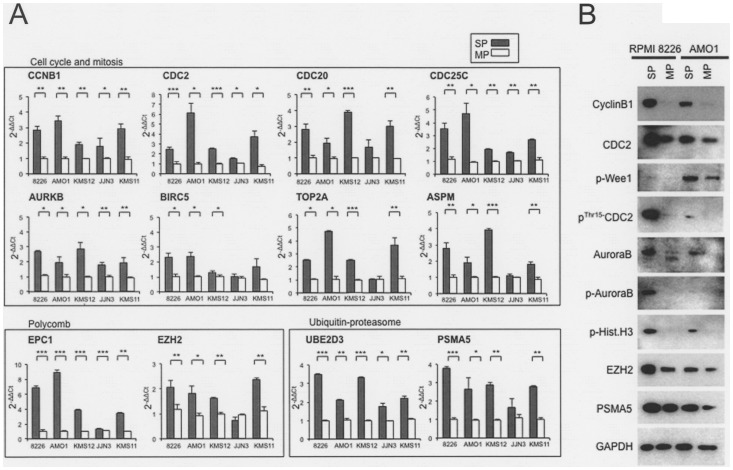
Real time quantitative PCR and western blot analyses of candidate markers of the SP in MM cells. (A). Real time quantitative PCR of cell cycle and mitosis related genes (*CCNB1,CDC2, CDC20, CDC25C, AURKB, BIRC5, TOP2A, ASPM),* Polycomb related genes (*EPC1, EZH2),* and ubiquitin-proteasome related gene (*UBE2D3* and *PSMA5)* against RPMI 8226, AMO1, KMS-12-BM, JJN3 and KMS-11 cells. Y-axis: gray and white bars depict 2^−ΔΔCt^ values for gene expression. Asterisks (*) indicate statistical significance: *0.01≤*P*<0.05, 0.001≤*P*<0.01, P*<0.001. Bars are means ± SD of three independent experiments. (B). Western blot analysis of Cyclin B1, CDC2, p-WEE1, p-CDC2, Aurora B, p-Aurora B, p-Hist.H3, EZH2, PSMA5 and GAPDH in SP and MP against RPMI 8226 and AMO1 cell lines.
